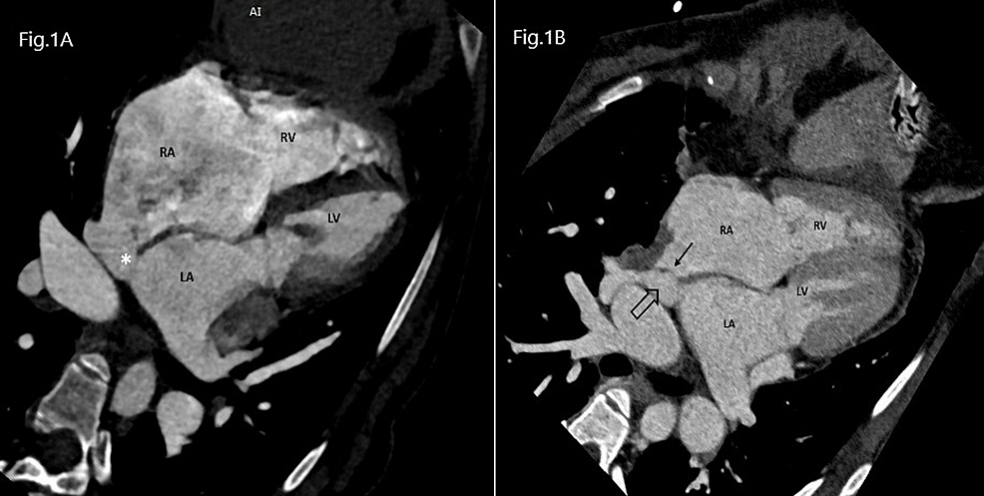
Section A: Contrast-enhanced CCT in axial four-chamber view showing a sinus venosus type defect in the interatrial septum (asterisk). Section B. Contrast-enhanced CCT in axial four-chamber view showing patch closure of atrial septal defect (thin black arrow) with pulmonary vein opening in the left atrium (thick black arrow) CCT: Cardiac Computed Tomography, RA: Right Atrium, LA: Left Atrium, RV: Right Ventricle, LV: Left Ventricle.
